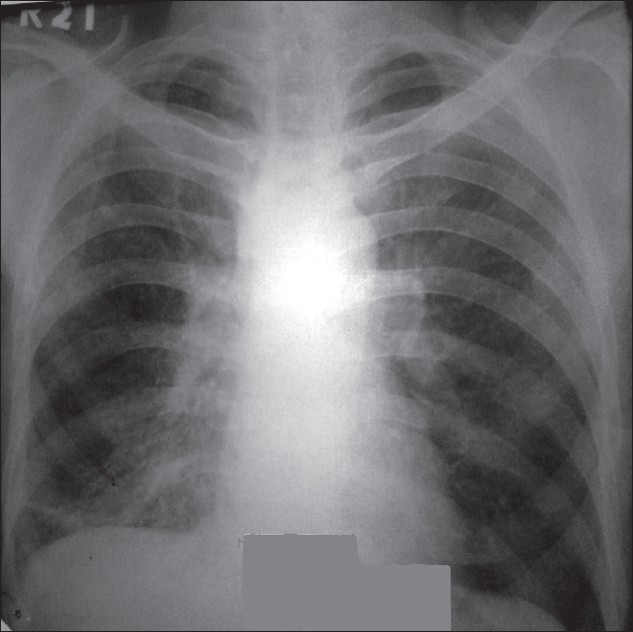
Chest radiograph shows right lower zone heterogenous shadows
